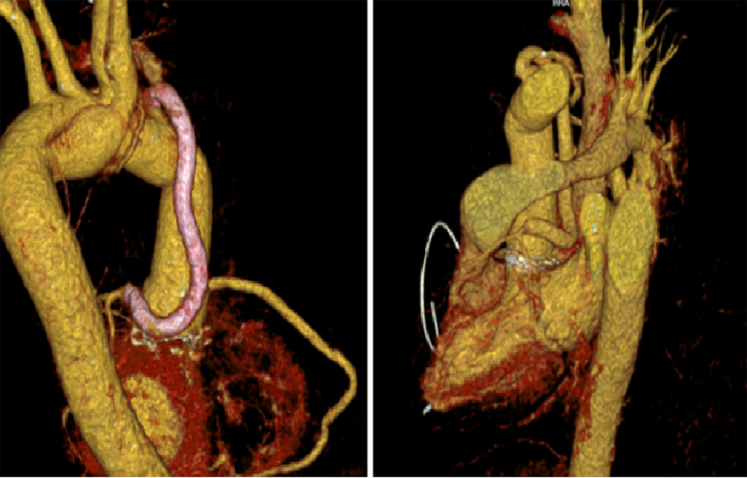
Three-Dimensional Cardiac CT Reconstruction Demonstrating Correct Positioning of a New Vascular Graft With a Mechanical Aortic Valve A hemi-Cabrol procedure was performed, consisting of a bypass conduit originating from the distal portion of the aortic prosthesis and anastomosed to the left main coronary trunk (highlighted in pink) ensuring unobstructed coronary perfusion. CT = computed tomography.
